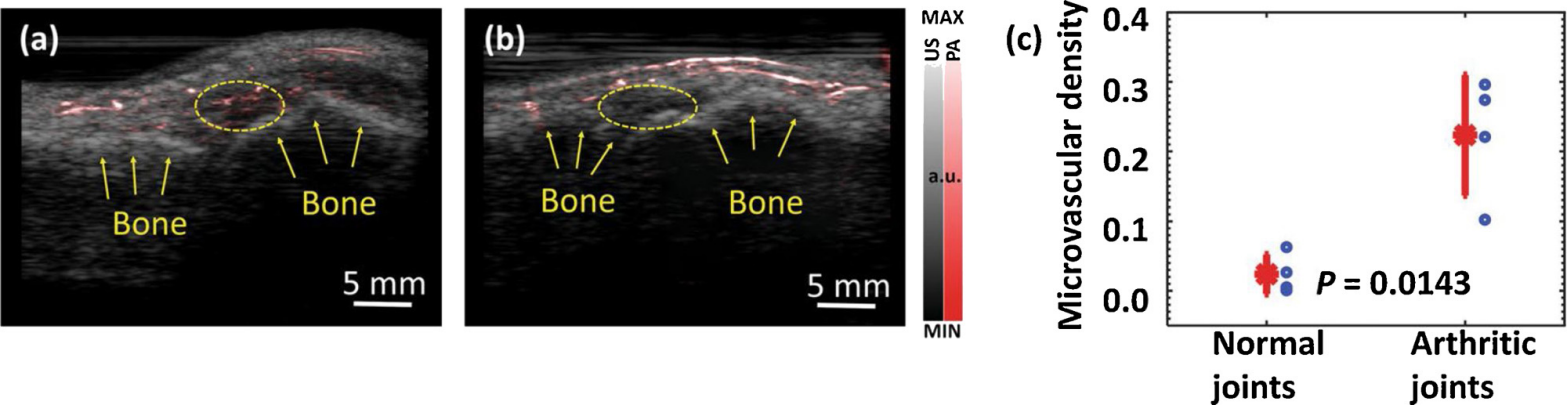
Co-registered photoacoustic and ultrasound images an arthritic (a) and healthy (b) human MCP joint. (c) Comparison of microvasculature density at normal and arthritic joints. Reprinted from Ref. [64].
